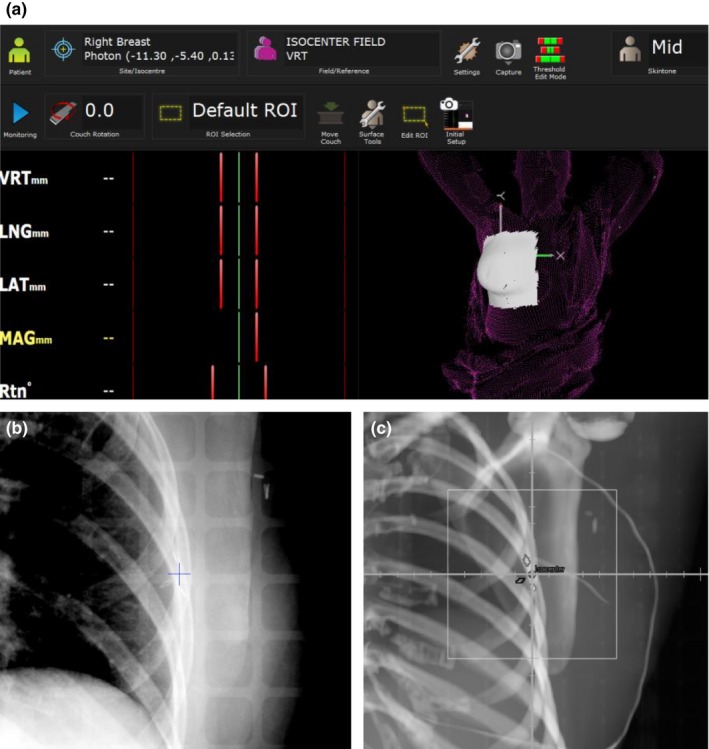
(a) Region of interest (ROI) using AlignRT for APBI with (b) associated example daily orthogonal x ray, and (c) digitally reconstructed radiograph.
